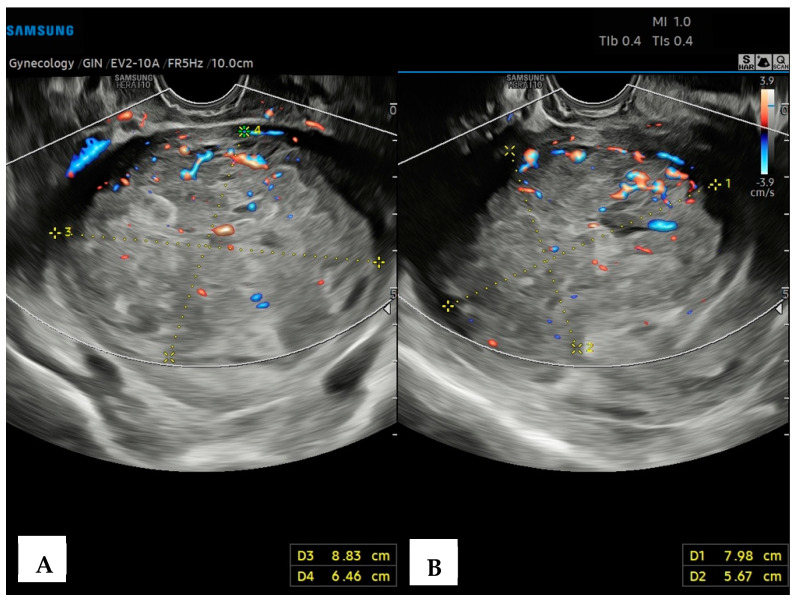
Transvaginal ultrasound with Color Doppler analysis. (A) Longitudinal plane; (B) axial plane showing a hypoechoic, heterogeneous uterine mass, without calcifications or acoustic shadows, measuring (L × H × W) 8.8 × 7.9 × 6.4 cm. The Color Doppler analysis reveals an abundant perilesional and intralesional vascularization.
